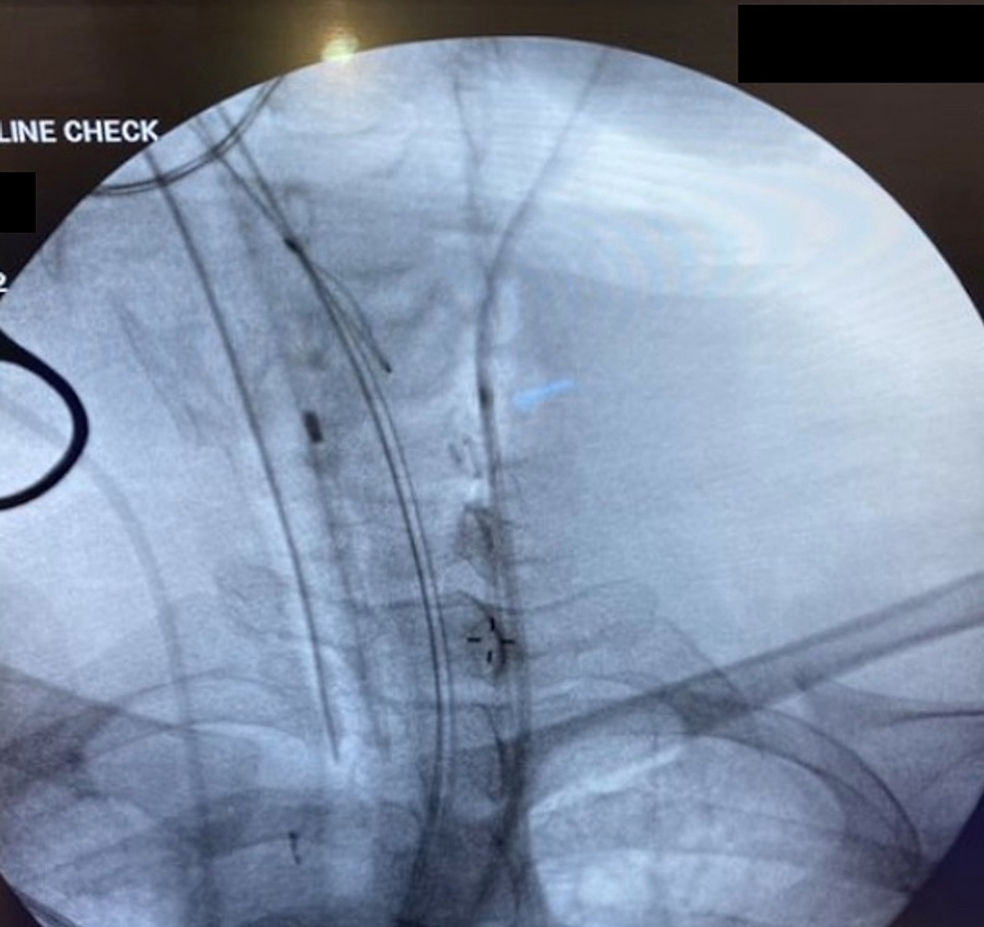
Anteroposterior X-ray Line Check
